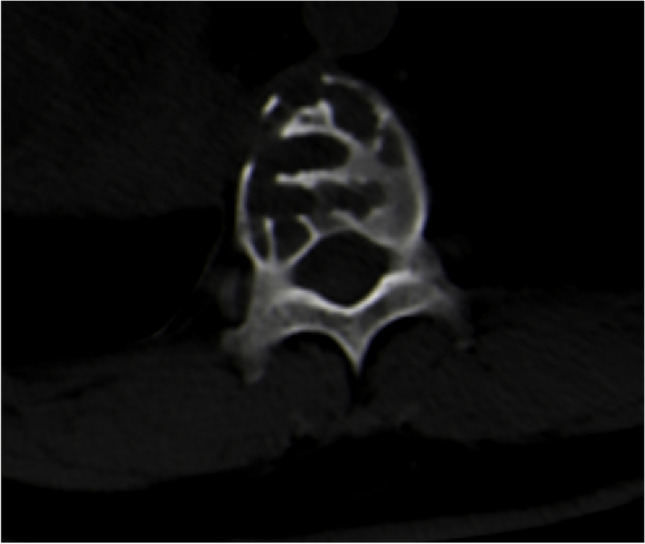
Plain CT scan showed different size bone destruction and dilated area with internal septum in the 11th thoracic vertebrae
